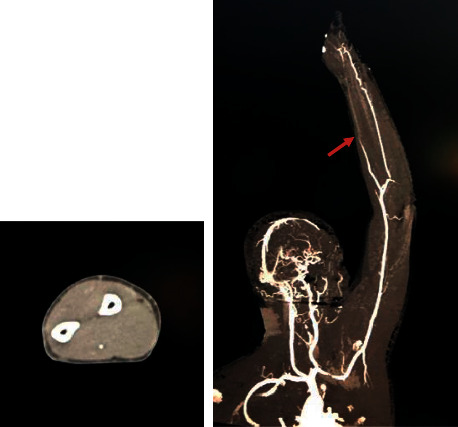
IV contrasted CT scan showing occlusion of the left radial artery at the level of the midforearm with retrograde filling at the level of the left rest.
